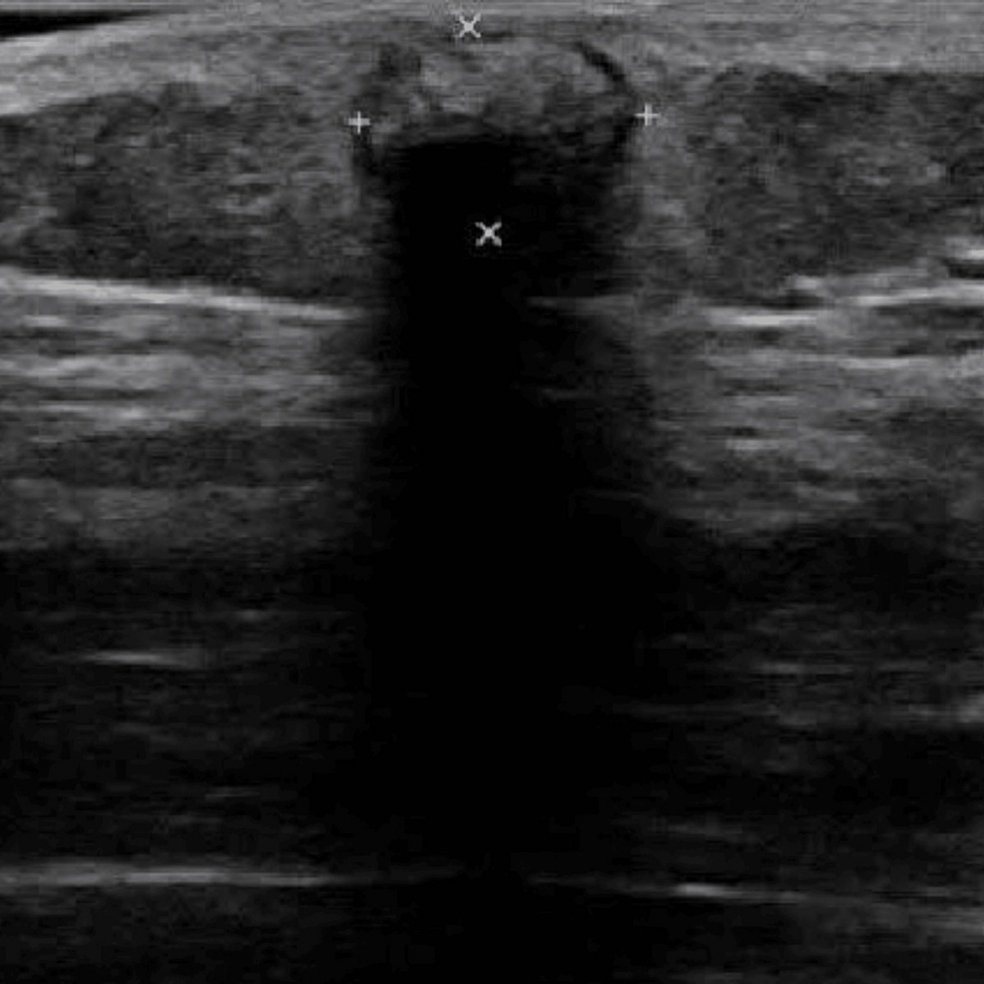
Ultrasound image showing hypoechoic region with posterior acoustic shadowing, indicative of foreign body or calcified structure within the tissue
